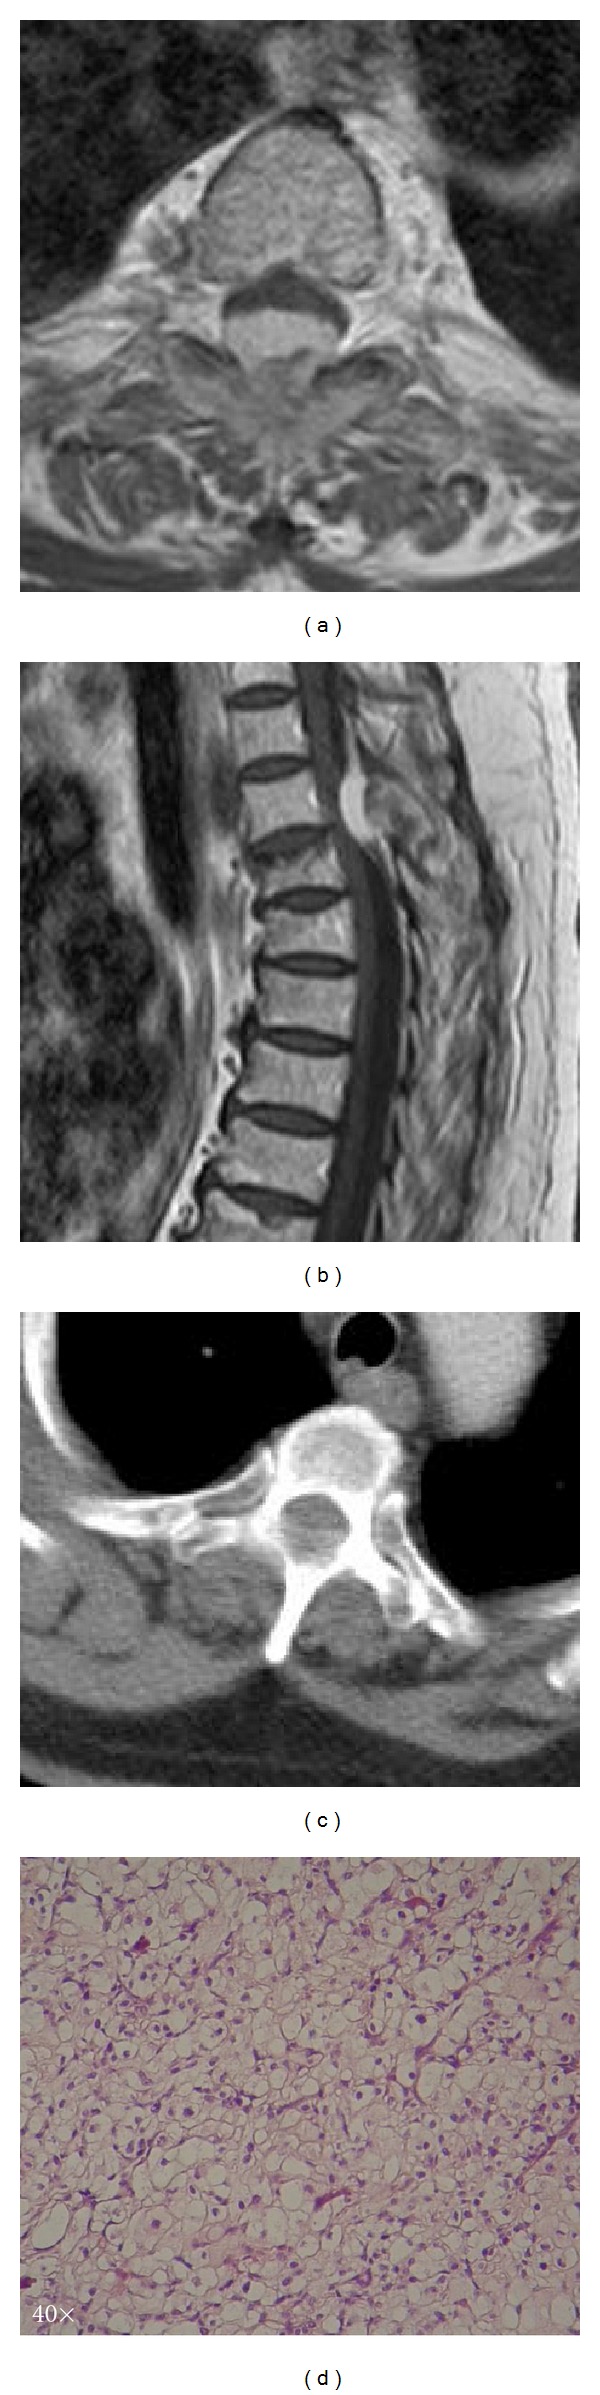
(a) Axial T2 weighted MR image of the lesion at Th3 level, demonstrating significant spinal cord compression; (b) Sagittal contrast enhanced T1 weighted MR image of the lesion, showing spinal cord compression at Th3-Th4 level; (c) Axial CT scan of the lesion at Th3 level, demonstrating spinal canal involvement, bony erosion, and paravertebral extension of the tumor; (d) H/E ×40 Clear cell chondrosarcoma characterised by tumor cells with variably clear cytoplasm.
